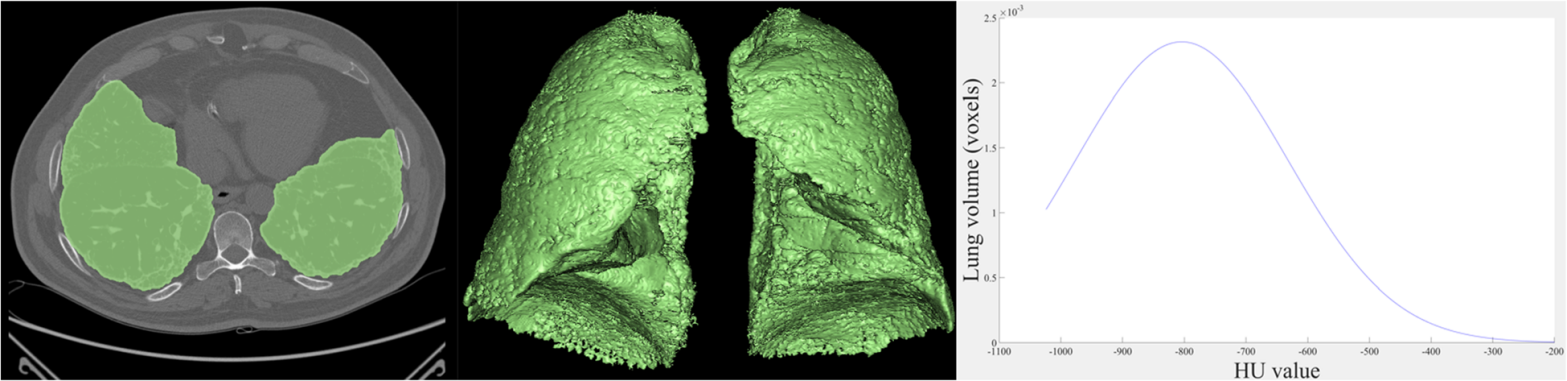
Example of lung parenchyma segmentation and CT-derived histogram
